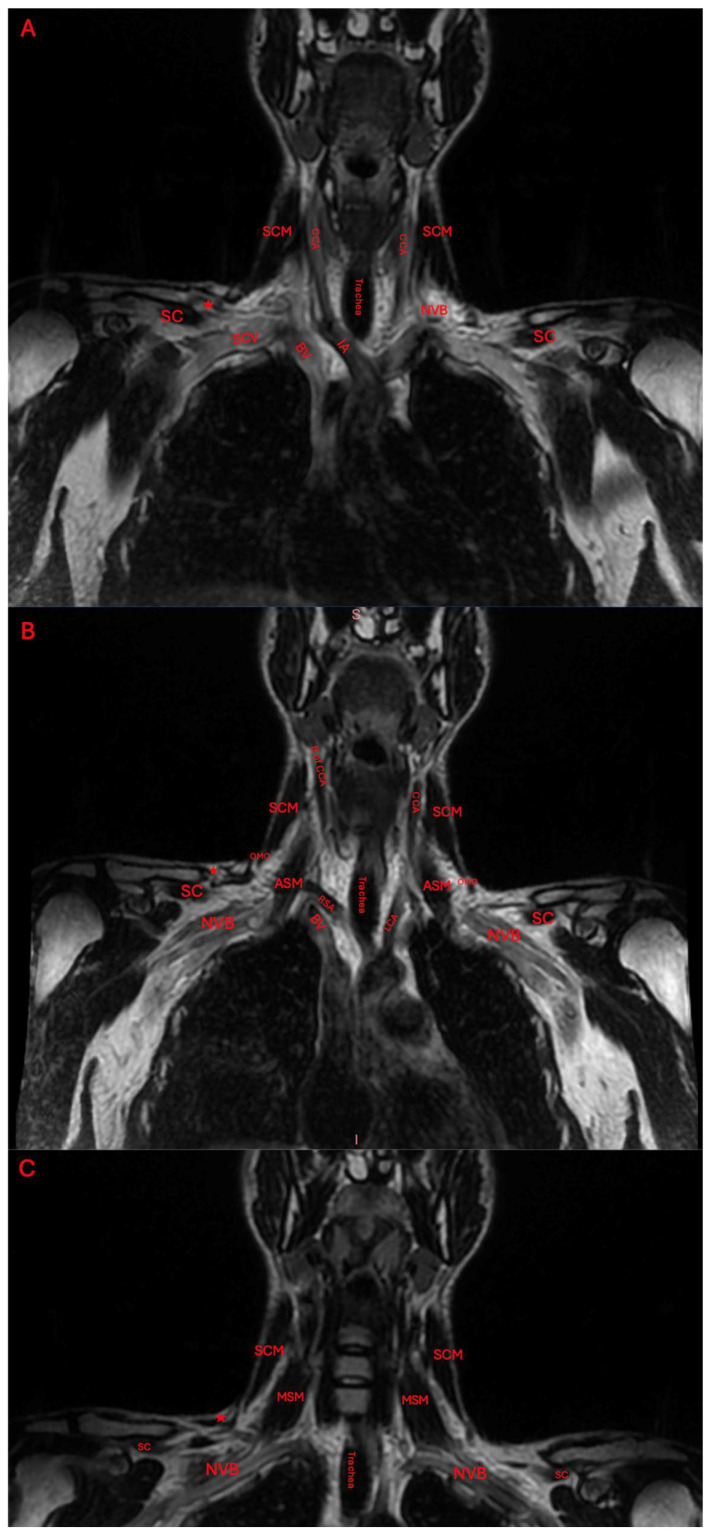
Coronal T2-weighted MRI imaging of the brachial plexus, neck, and shoulder moving from anterior to posterior (A–C). The red star marks the insertion of the levator claviculae. The other abbreviations are the bifurcation of the carotid artery (B of CCA), left common carotid artery (LCA), innominate artery (IA), sternocleidomastoid (SCM), right subclavian artery (RSA), anterior scalene muscle (ASM), middle scalene muscle (MSM), brachiocephalic vein (BV), omohyoid (OMO), subclavius muscle (SC), and neurovascular bundle (NVB).
